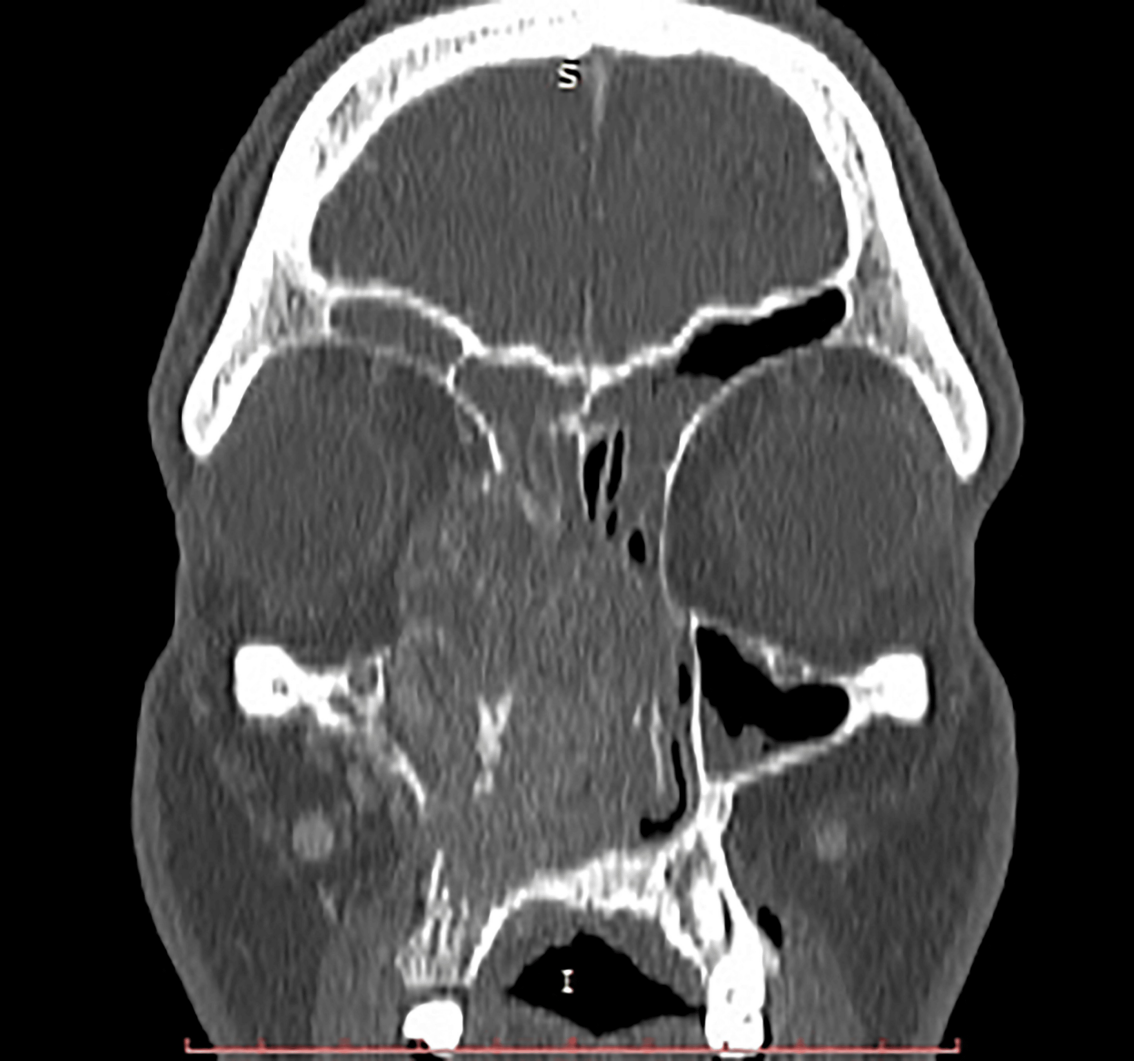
Sagittal view of CT scan of the head The right side of the nasal cavity was filled with tumor mass, which displaced the septum to the left.
